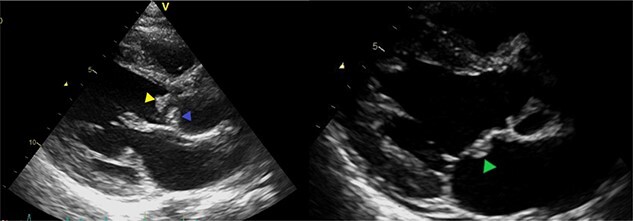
Echocardiography showing vegetations at the aortic and mitral valves (anterior aortic valve = yellow arrow; posterior aortic valve = blue arrow; anterior mitral valve leaflet = green arrow).
